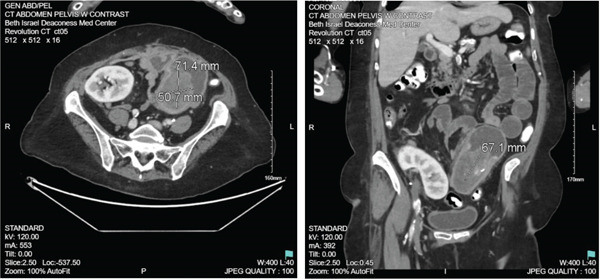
Contrast‐enhanced CT images of the abdomen showing a jejunal mass with features of partial small bowel obstruction.
